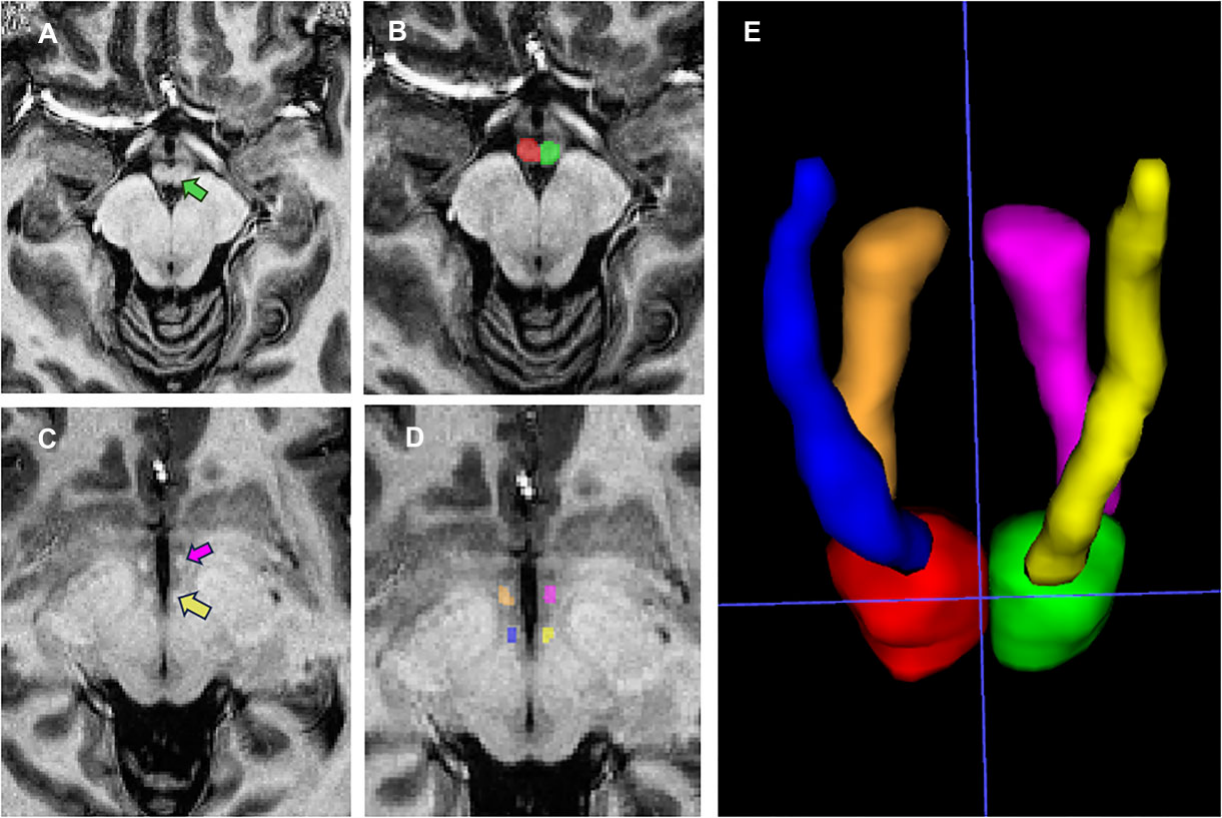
MB, MTT, and PF structures on MRI. (A–D) Green denotes left MB; pink denotes left PF; yellow denotes left MTT. Red, orange, and blue regions denote the right MB, PF, and MTT, respectively. (E) 3D view of the MB, MTT, and PF.
